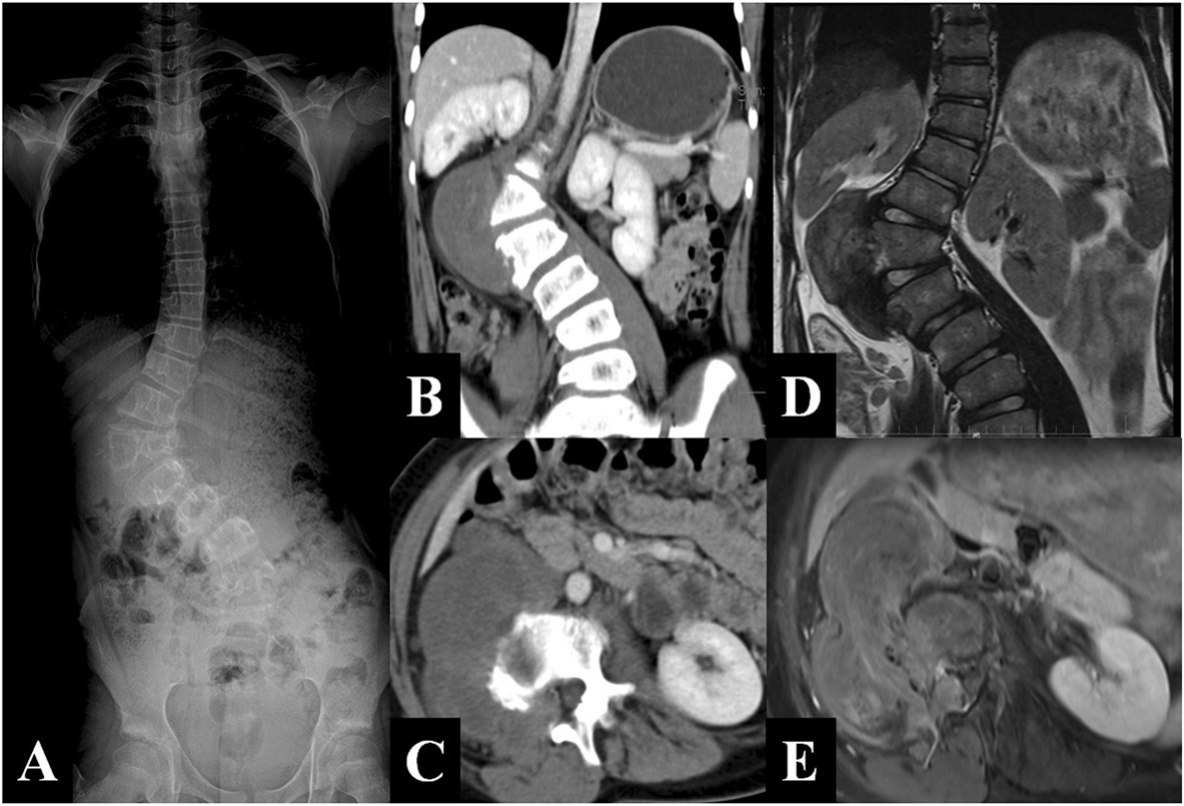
a The lower segment of the thoracic and lumbar spine existed a right tumefied thoracolumbar curve surrounding the first lumbar body with a Cobb angle of 33.7° and Ferguson angle of 69.4°. b, c Axial CT scans and coronary multi-plane reorganization demonstrated a right paravertebral irregular soft tissue mass with low and nonhomogeneous density, as well as uneven strength from the T_12_ to L_2_ vertebrae. The tumor grows through L_1/2_ right intervertebral foramen lesions to spinal canal. The intervertebral foramen becomes larger, and the right side of L_2_ vertebrae with irregular bone becomes corroded damage. d, e Axial enhanced MRI scan demonstrated the tumor is less homogeneous reinforcement, grows through L_1/2_ right intervertebral foramen lesions to spinal canal. The spinal shift to the left side due to cord compression
